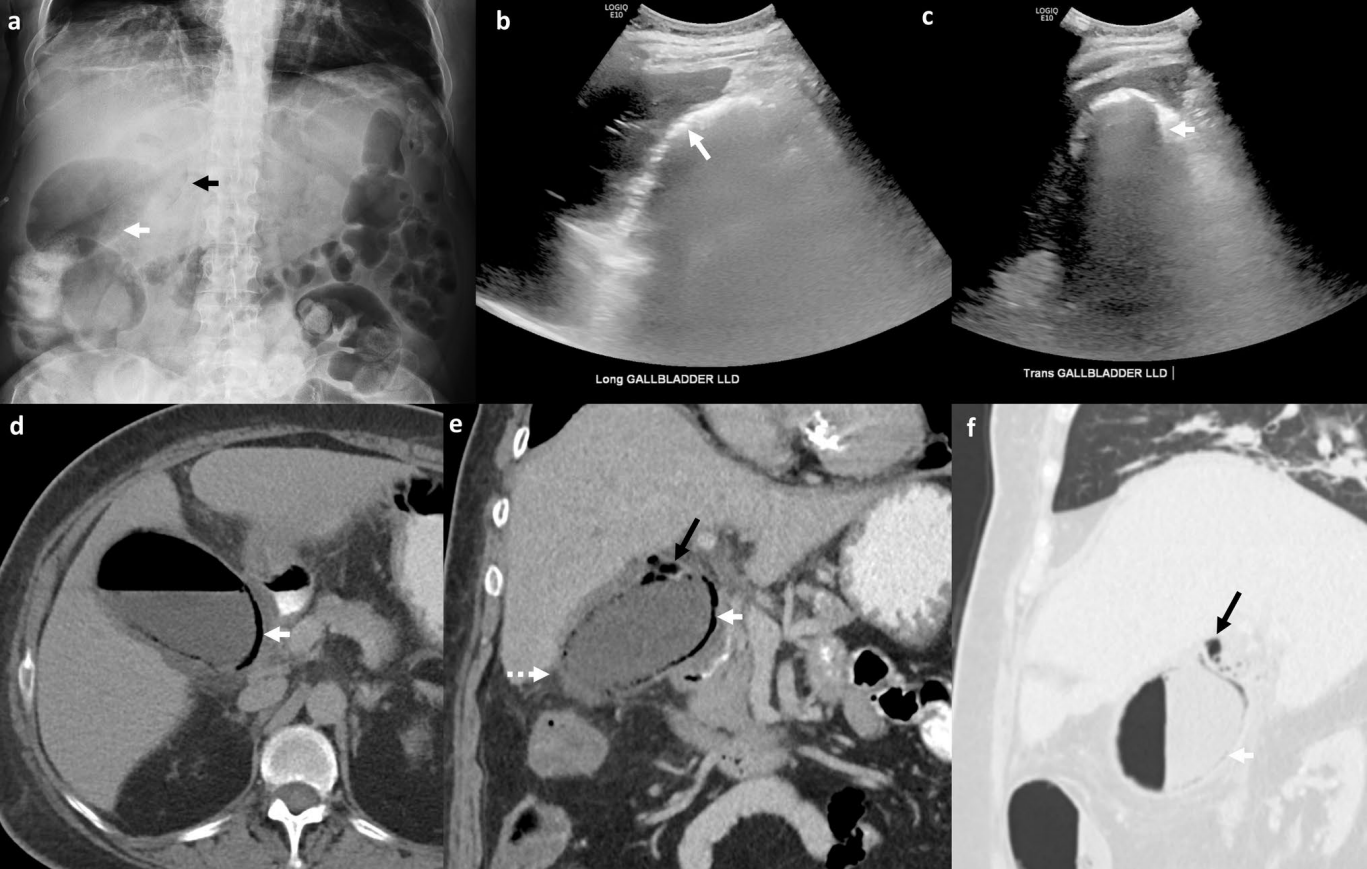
Emphysematous cholecystitis. A 54-year-old with diabetes mellitus and end-stage renal disease presented with right upper quadrant pain and sepsis. Abdominal radiograph a shows air in the right upper quadrant within the expected location of the gallbladder (white arrow, a) and abnormal curvilinear air in the right upper quadrant (black arrow,a). Longitudinal (b) and transverse (b) US images show curvilinear echogenic air foci in the gallbladder wall with dirty posterior acoustic shadowing (white arrows, b, c). CT images d–f show gallbladder wall thickening with air in the gallbladder wall (solid white arrows, d–f), air in the cystic duct (black arrows,e, f), and pericholecystic inflammation (dotted white arrow, e)
